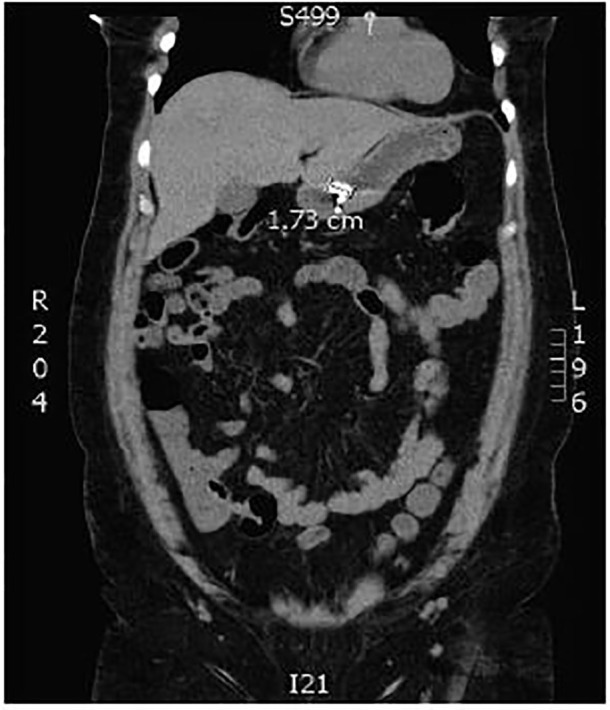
Coronal view from computed tomography of the abdomen and pelvis noting the foreign body in the distal stomach.
